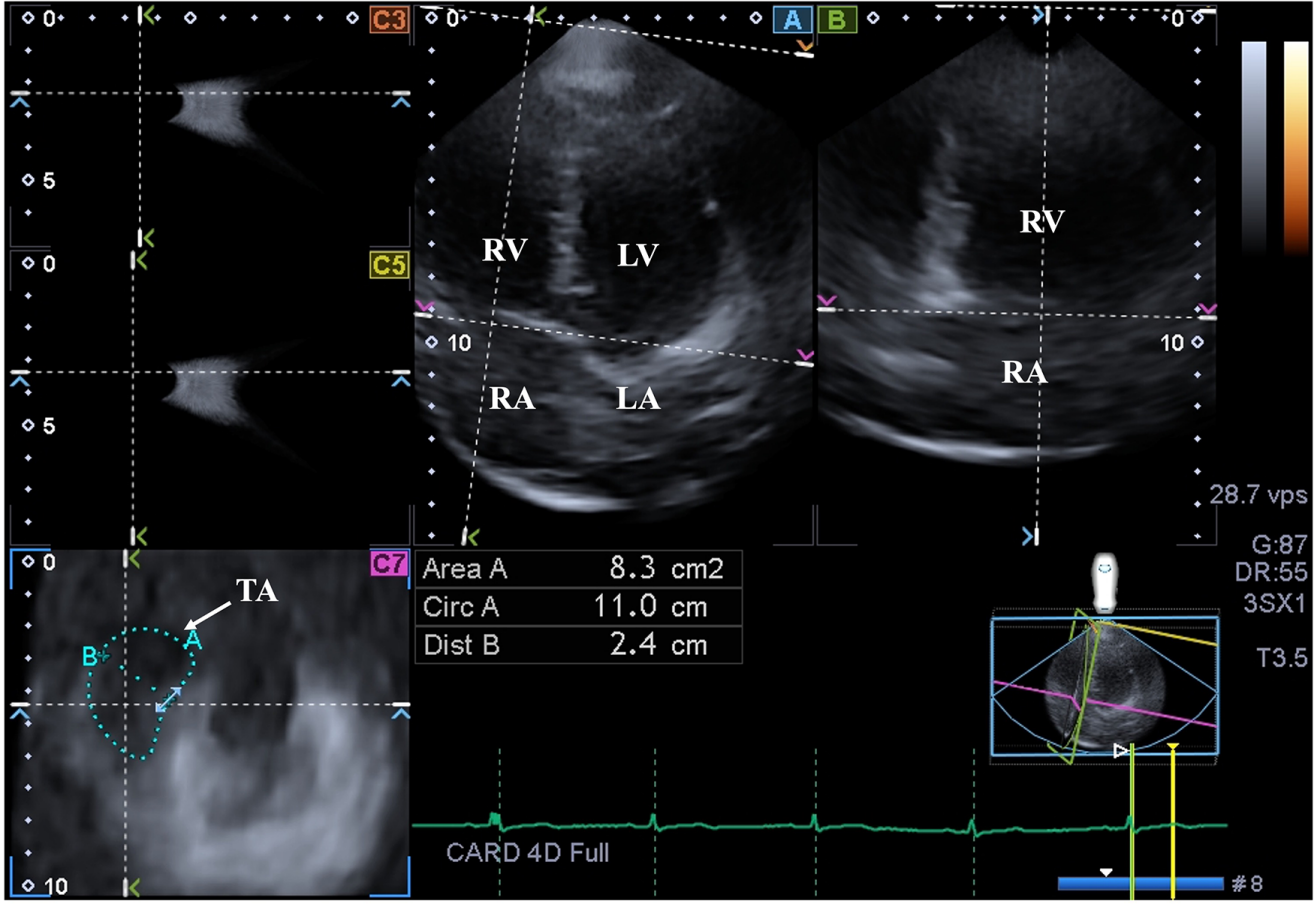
Three-dimensional (3D) speckle-tracking echocardiographic assessment of the tricuspid annulus in a healthy subject: apical longitudinal four-chamber (A) and two-chamber views and cross-sectional view (C7) of the tricuspid annulus optimalised on (A) and (B) images. White arrow indicate plane of the tricuspid annulus. LA, left atrium; LV, left ventricle; RA, right atrium; RV, right ventricle; Area, tricuspid annular area; Circ, tricuspid annular perimeter; Dist, tricuspid annular diameter.
